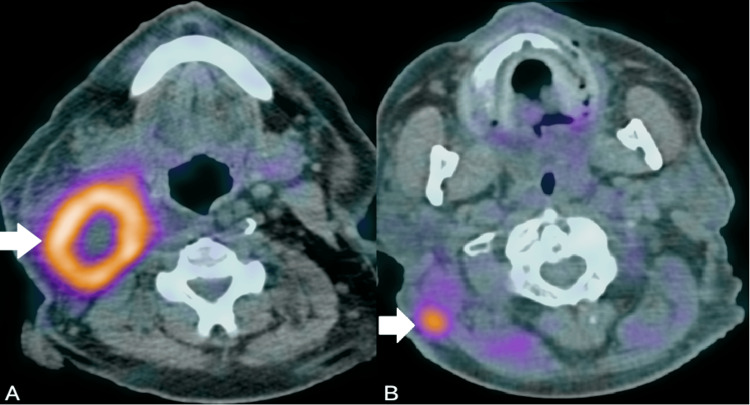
FDG PET scan of the patient at initial presentation. FDG PET scan of the patient at initial presentation demonstrating a hypermetabolic right cervical lymph node block, suspicious for malignancy (white arrow - panel A). FDG PET scan of the patient at recurrence, displaying a hypermetabolic right sternocleidomastoid lesion, suspicious of cancer recurrence (white arrow - panel B). FDG PET: Fluorodeoxyglucose Positron Emission Tomography
